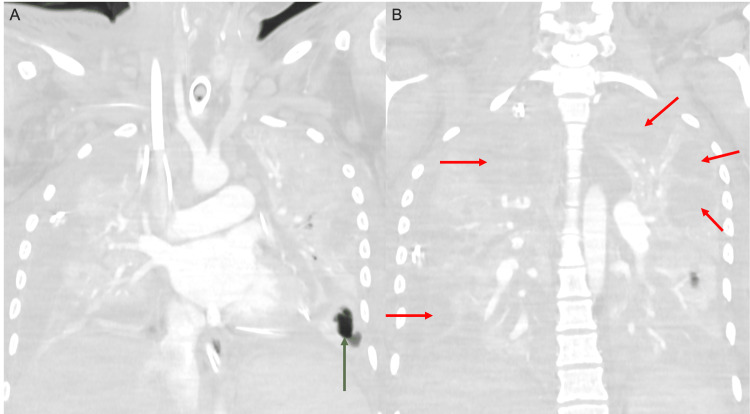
Coronal contrast-enhanced CT images of the chest in the lung window (A, B) demonstrate near-complete opacification of all lobes of the lungs bilaterally, with a small focal area of aeration involving the left lower lobe (green arrow in A). Multiple cystic collections are also present throughout the lungs (red arrows in B).
